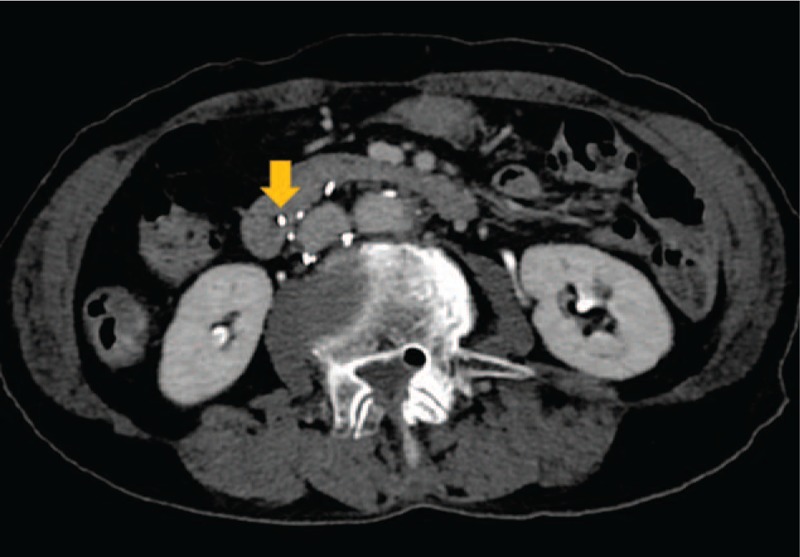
A 74-year-old-woman with abdominal and back pain, nausea and vomiting. Computed tomography shows one of the struts (arrow) of the inferior vena cava (IVC) filter has migrated through the IVC wall into the duodenum.
